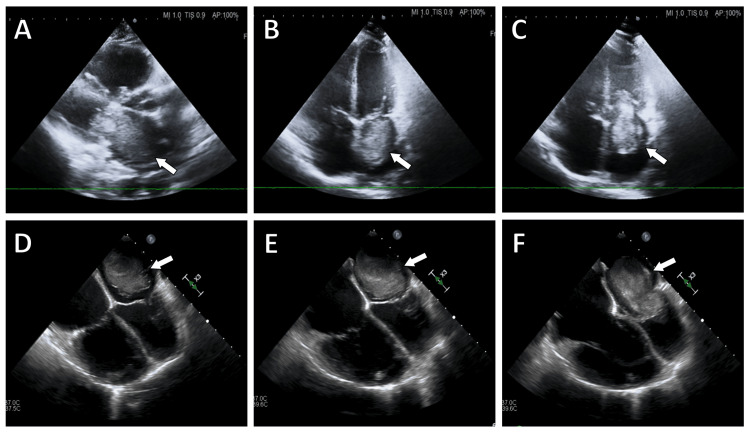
Echocardiographic images showing the left atrial myxoma. Echocardiographic images showing the left atrial myxoma (arrow). Transthoracic echocardiography (TTE) short-axis view (A) and four-chamber view in the systolic (B) and diastolic (C) phases. Transesophageal echocardiography (TEE) images demonstrating the movement of the myxoma in the systolic (D, E) and diastolic phases (E).
